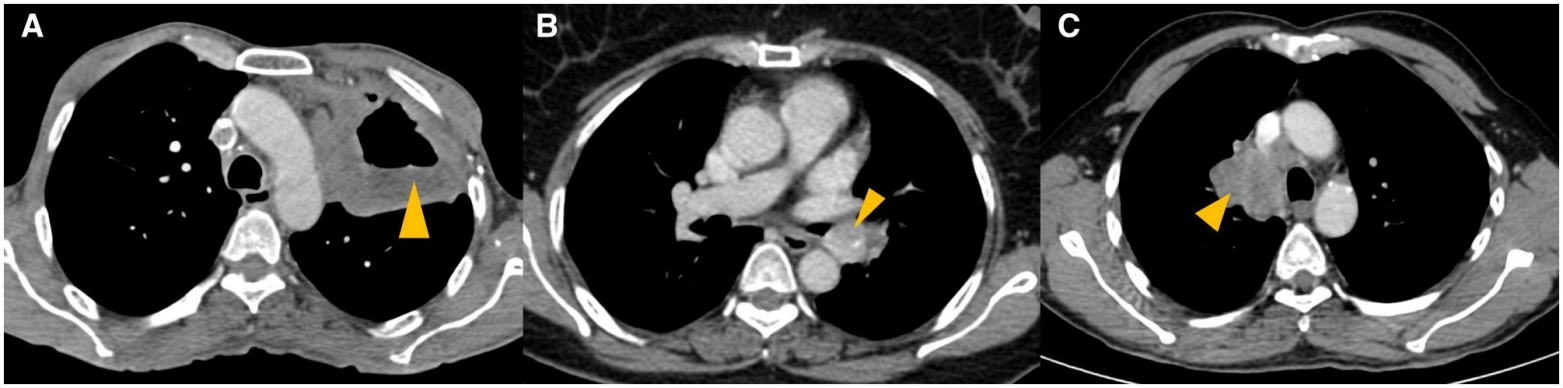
(A-C) Contrast enhanced computed tomography (CECT) of types of lung cancer. (A) CECT thorax (mediastinal window) shows cavitation within a solid left upper lobe lung mass (arrowhead), in this 70 years old male (with history of smoking) with biopsy proven squamous cell carcinoma. (B) CECT thorax (mediastinal window) of a 45 years old male with biopsy proven typical carcinoid, shows an enhancing endobronchial lesion in left main bronchus (arrowhead). (C) CECT thorax (mediastinal window) in this 75 years old male (with history of smoking) with biopsy proven small cell lung cancer, shows centrally located, right suprahilar mass conglomerating with, and inseparable from right paratracheal nodes (arrowhead).
